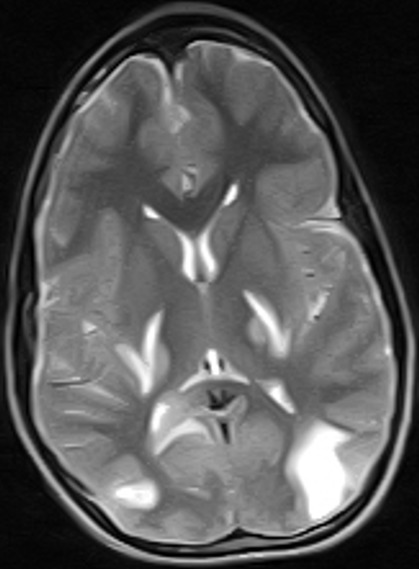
(MRI post treatment) is a T2 w axial sequence showing residual leisons
